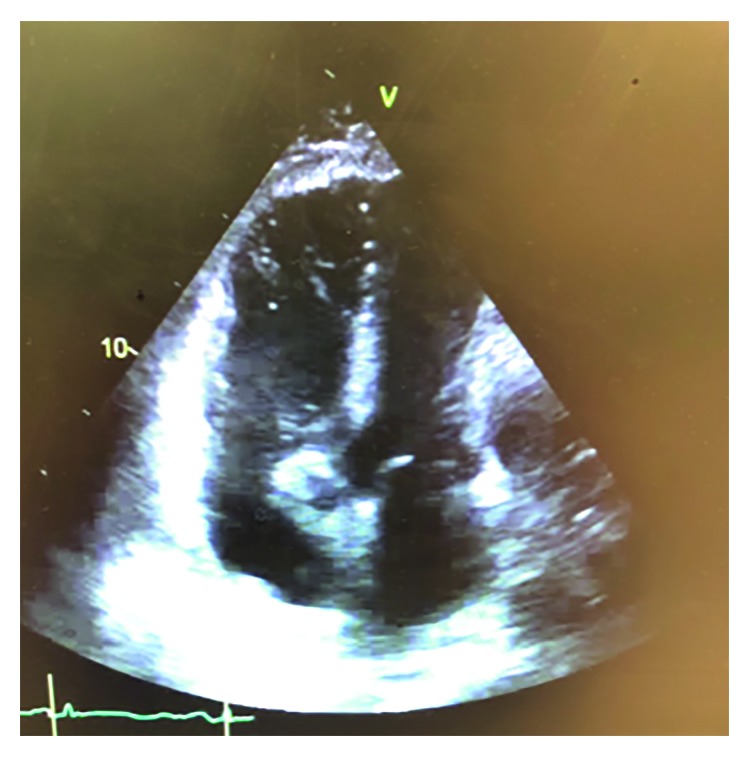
2D echocardiogram image of cardiac tamponade with right ventricular diastolic collapse and a large fibrinous exudative pericardial effusion.
